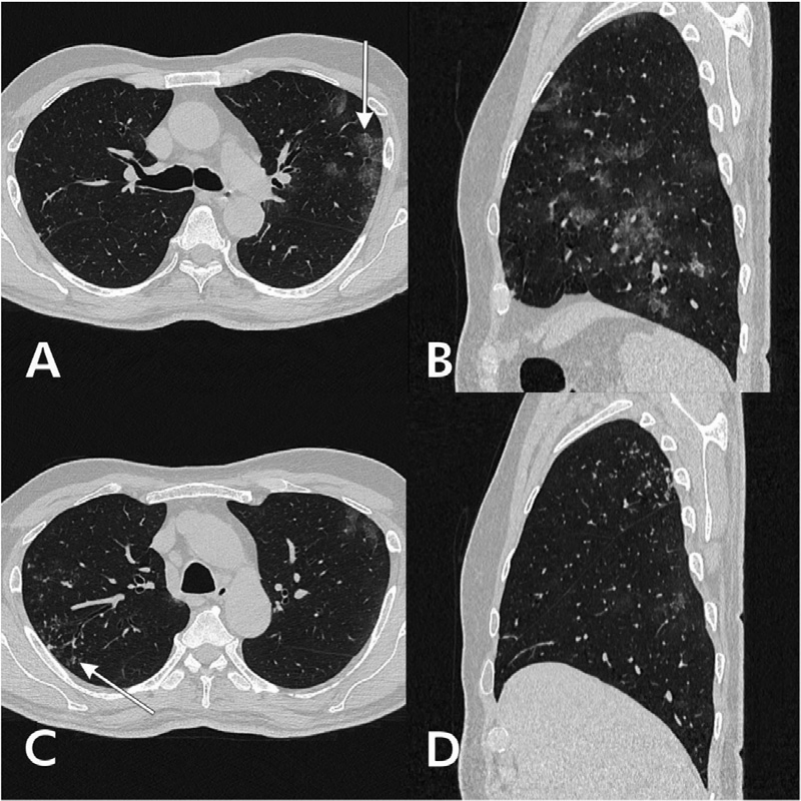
A-D: (A) Axial HRCT thorax shows rounded subpleural GGOs in left upper lobe (white arrow). (B) Sagittal HRCT thorax of the left lung shows multifocal GGOs. (C) Axial HRCT thorax shows multiple tiny nodular opacities in right upper lobe (white arrow). (D) Sagittal HRCT thorax of the right lung shows tiny nodular opacities in upper lobe and patchy GGOs in lower lobe.
